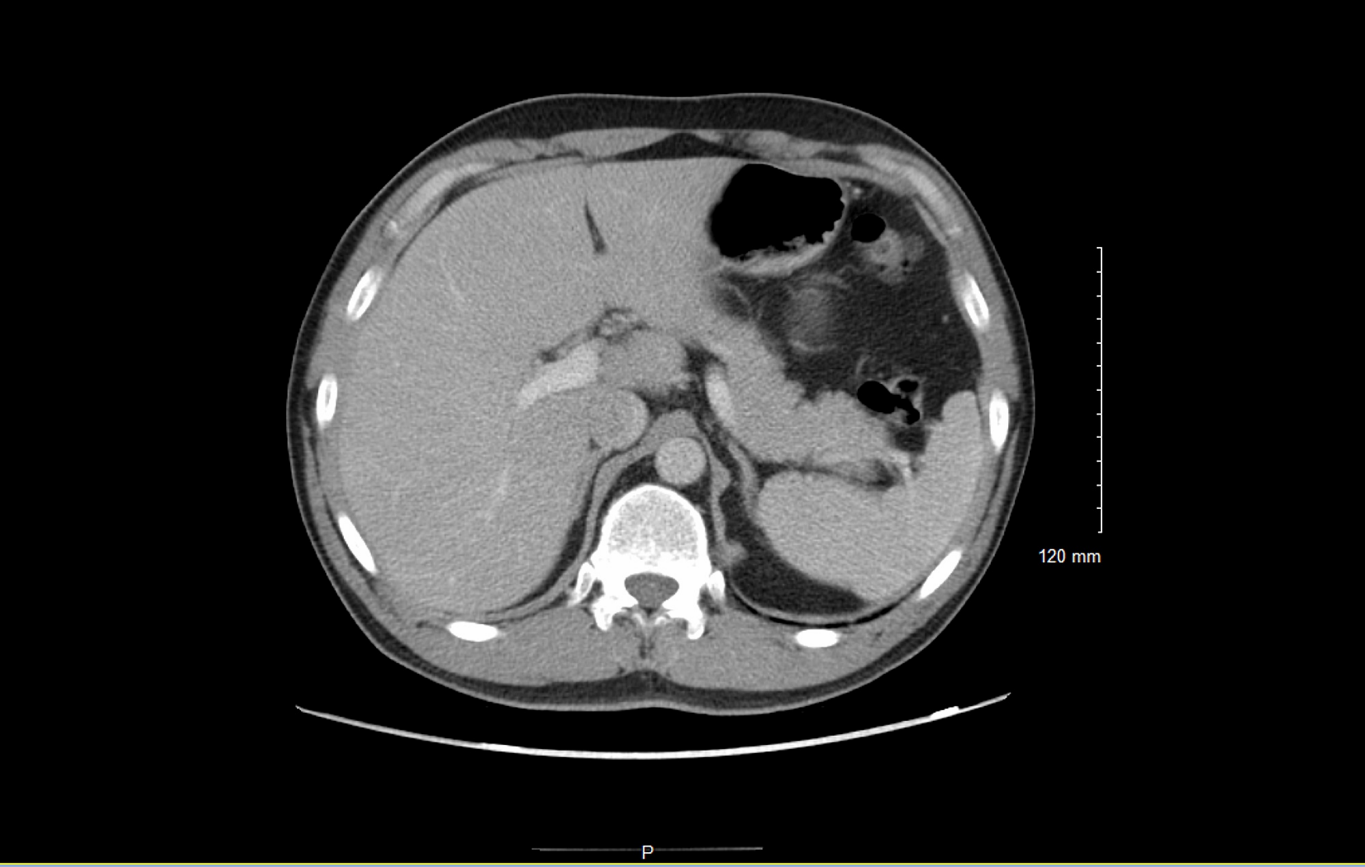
Repeat CT abdomen and pelvis showing resolution of collection with no new abscess
